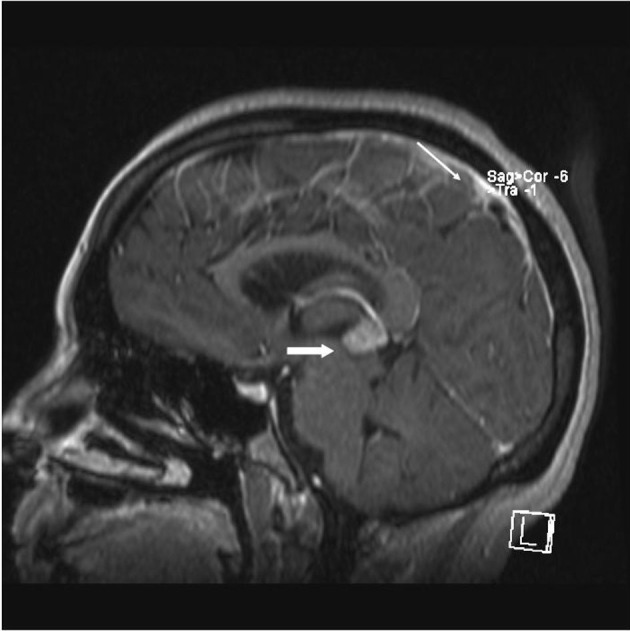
MRI brain (saggital) reveals obstructive hydrocephalus caused by an enhancing pineal gland mass (large arrow) and leptomeningeal carcinomatosis (LC) (small arrow).
